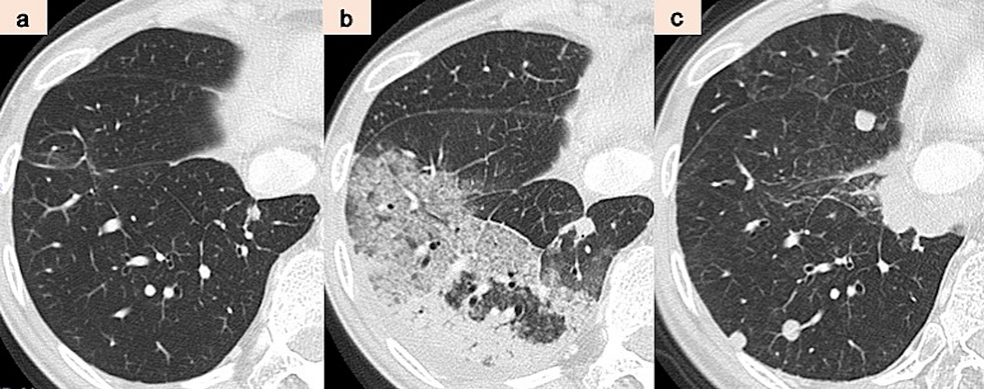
(a-c) Imaging findings on CT. CT: computed tomography
